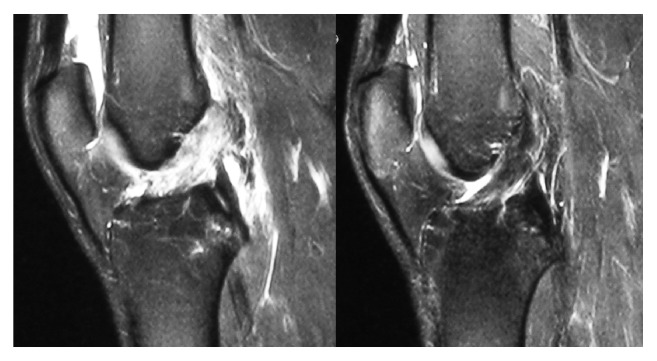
Initial and follow-up MRI six months after treatment with the ACL-Jack brace.
